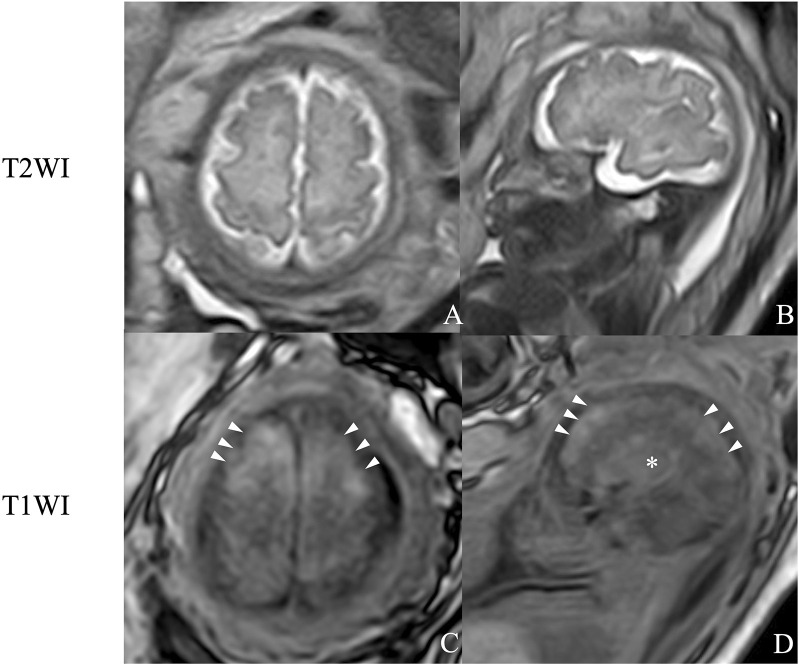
Cortical dysplasia MRI characteristics on fetal brain MRI, Cortical dysplasia is more easily detected on T1WI than on T2WI. (A, B) T2WI shows suspicious lesions in the fetal cortex and white matter; (C, D) T1WI reveals extensive cortical dysplasia in the fetus. The white arrow represents the cortical dysplasia lesion. The asterisks indicate numerous lesions also present in the basal ganglia region.
